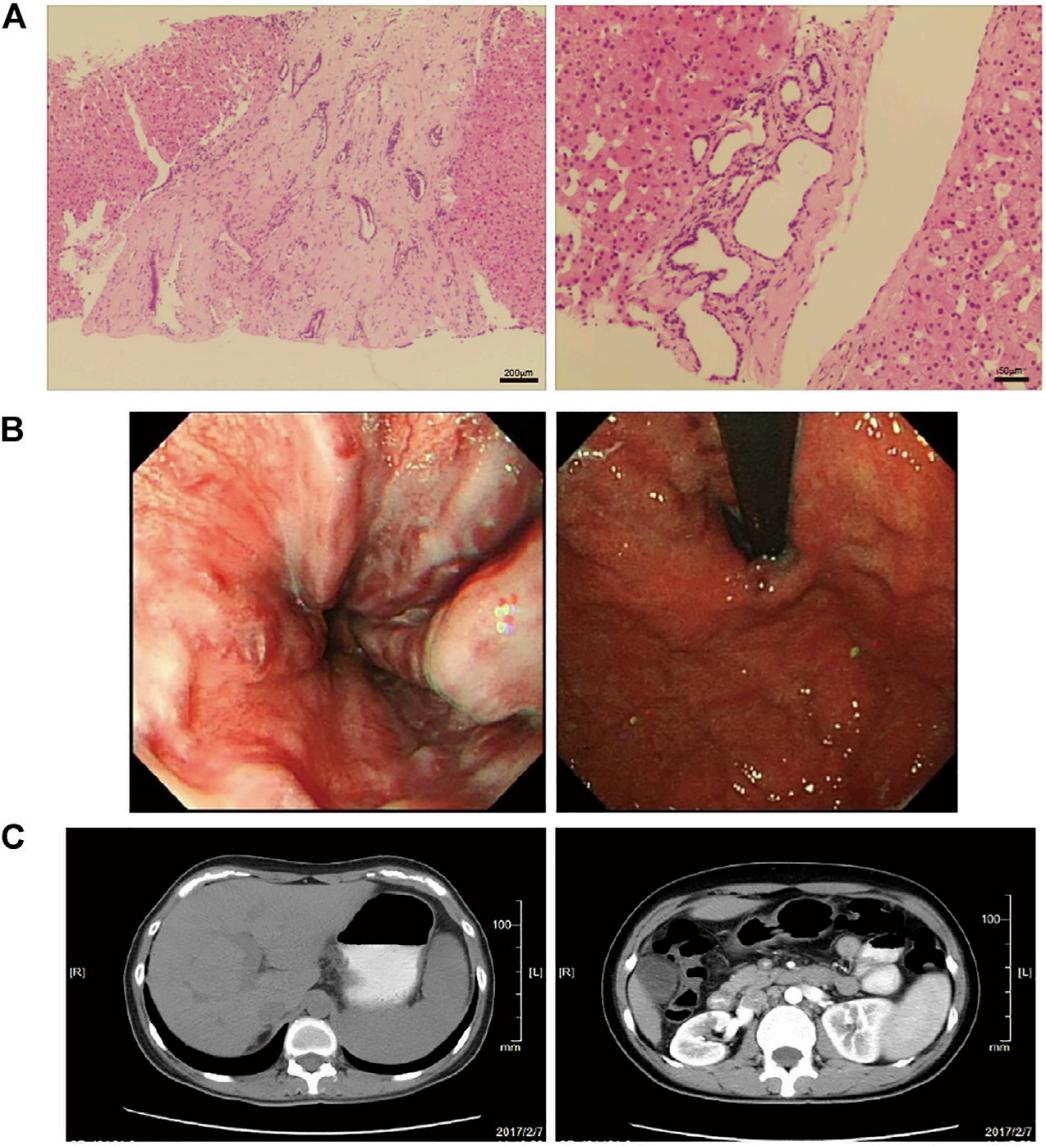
The pathological and clinical presentation of one CHF patient with NCPH. (A) The left panel shows diffuse fibrosis in the liver. The right panel shows a small bile duct hamartoma. (B) Endoscopy showed esophageal varices (left) and gastric varices (right). (C) The abdomen CT images show slight dilatation of the intrahepatic bile duct and splenomegaly (left), and multiple renal cysts in the bilateral kidneys (right).
